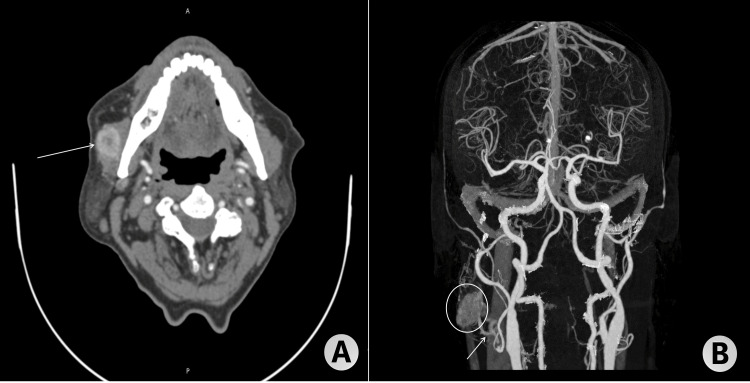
Imaging findings of the right parotid-masseteric lesion (A) Axial CECT demonstrating a well-defined, hyperenhancing mass in the right parotid-masseteric region (arrow), consistent with a hypervascular lesion. (B) Three-dimensional CT angiographic reconstruction showing a highly vascular mass (circle) with prominent arterial supply from branches of the external carotid system (arrow), initially suggestive of a high-flow vascular malformation. CECT: contrast-enhanced CT
